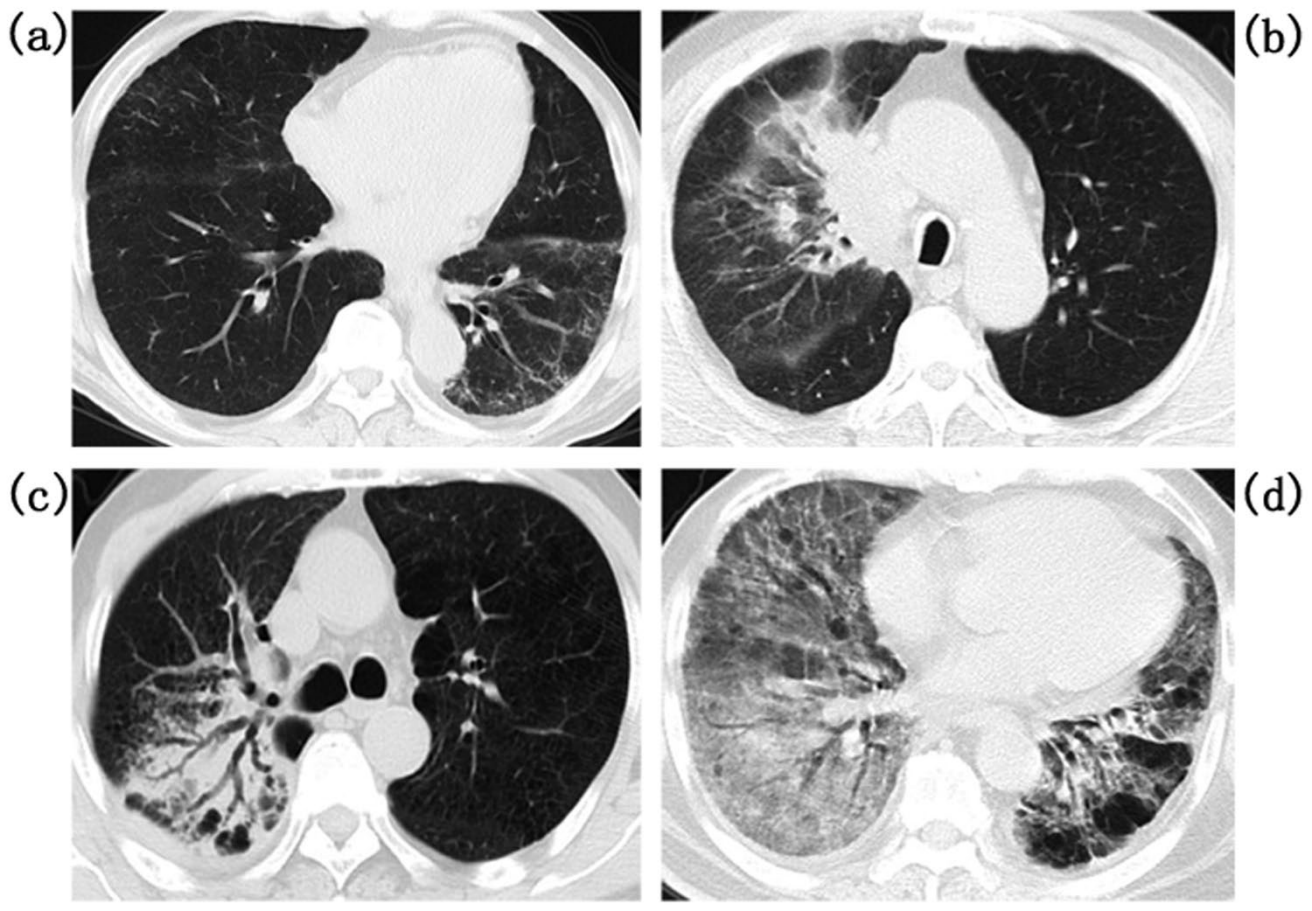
Radiation pneumonitis (RP) in computed tomographic (CT) images: (a) Grade 1, (b) Grade 2, (c) Grade 3, and (d) Grades 4–5.
